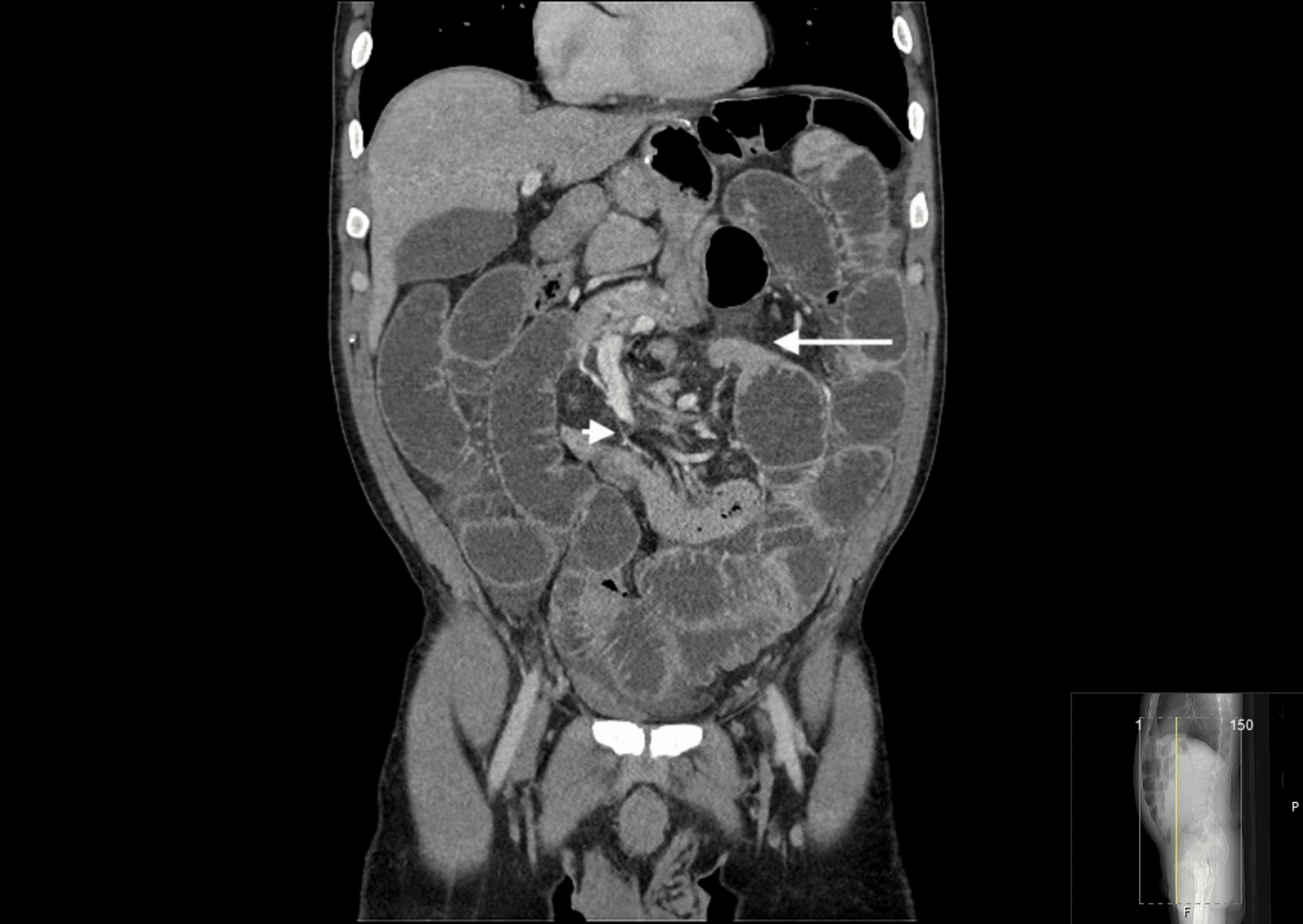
Preoperatory CT scan Short arrow: Mesenteric twisting; Long arrow: Distended bowel loops with a transition point close to the root of the mesenterium
